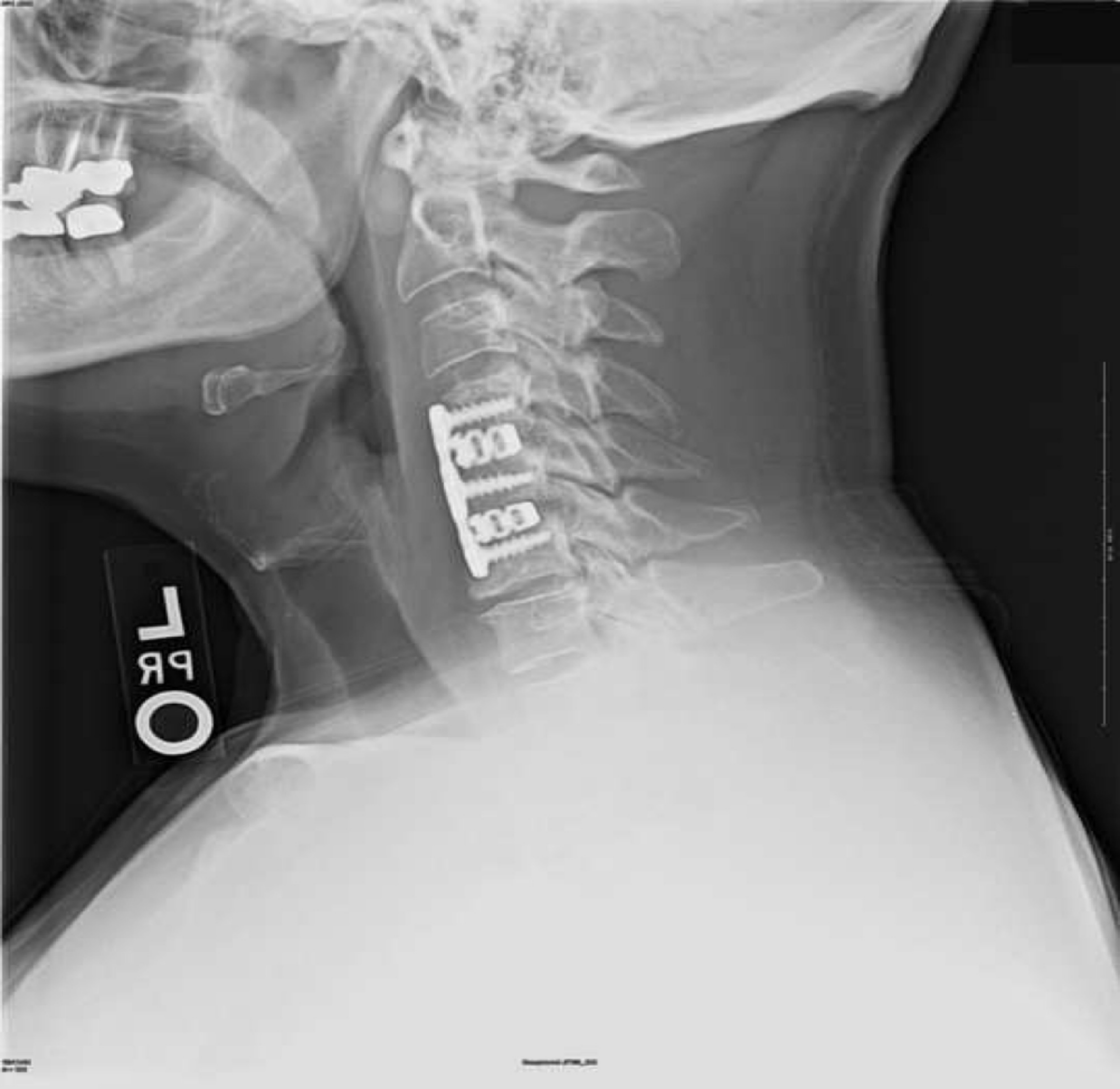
Lateral X-ray at four months showed improved alignment, resection of posterior osteophytes, and early graft incorporation
